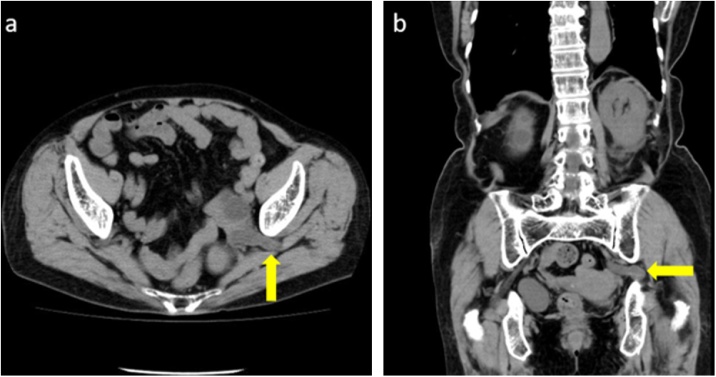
Unenhanced abdominal computed tomography scan revealed invagination of the left ureter into the left sciatic foramen (arrow). a. axial view, b. coronal view.
